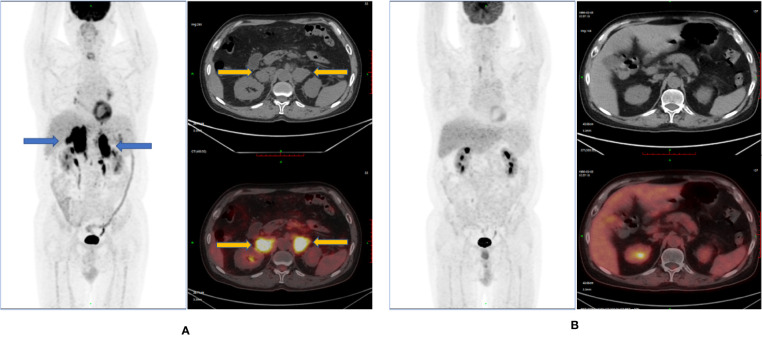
Remission status r/r DLBCL after anti-CD19 CAR-T-cell therapy and sirolimus. (A) Full-body ^18^FDG-PET scan pre- anti-CD19 CAR-T-cell therapy. (B) full-body ^18^FDG-PET scan post- anti-CD19 CAR-T-cell therapy.
